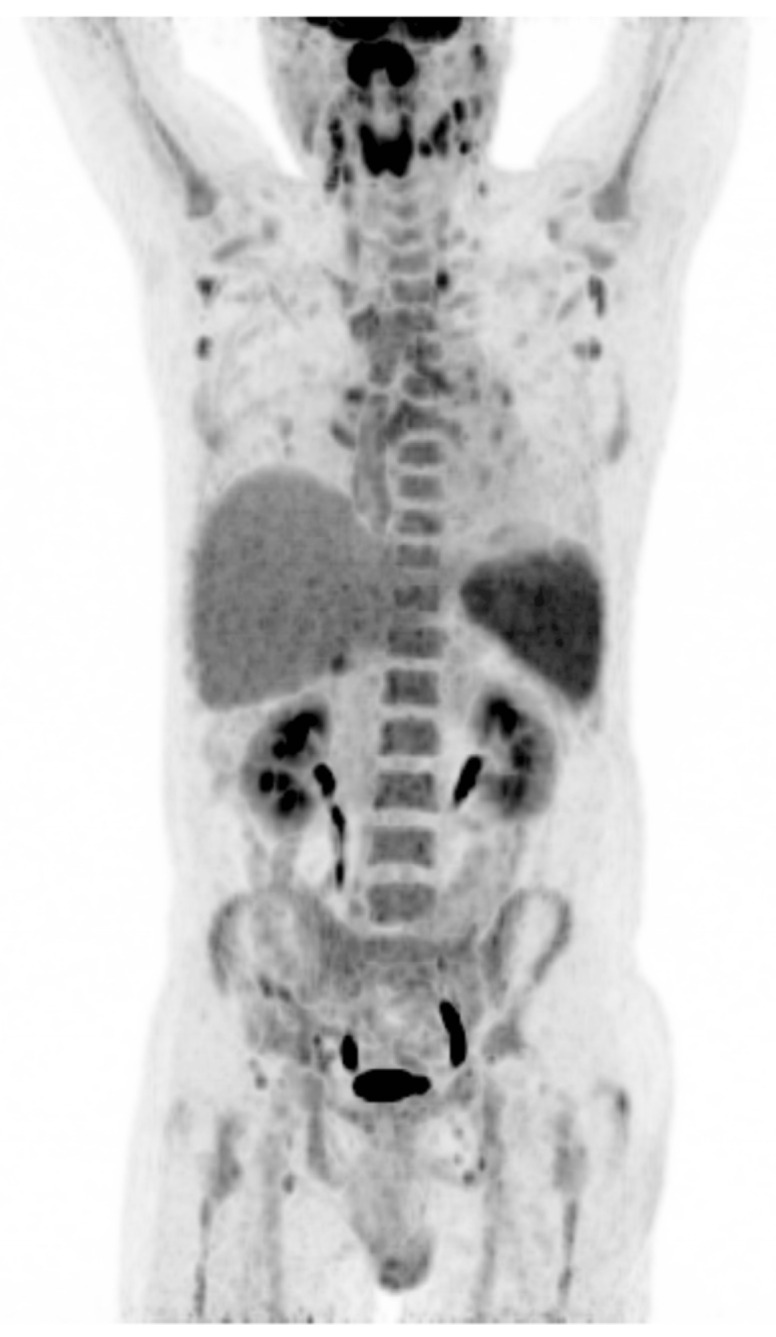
18F-FDG PET/CT scan (coronal maximum intensity projection) was ordered to evaluate fever of unknown origin. Intense uptake in the lymphatic tissue of the Waldeyer’s pharyngeal ring, the spleen, peripheral lymph nodes, and bone marrow are depicted consistent with a diagnosis of primary EBV infection in context of characteristic laboratory findings and not lymphoma. 18F-FDG = 18-fludeoxygluose; PET/CT = positron emission tomography/computed tomography.
